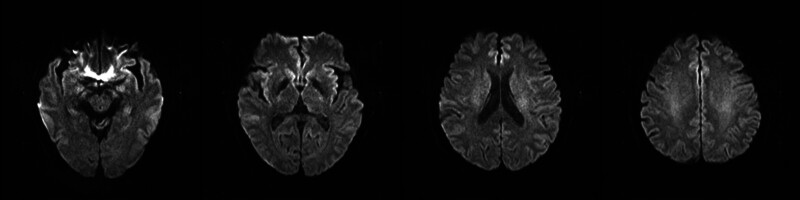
Brain diffusion-weighted MRI were obtained one month later. Note that the abnormal signals shown in Figure 1. Completely disappeared. MRI = magnetic resonance imaging.
